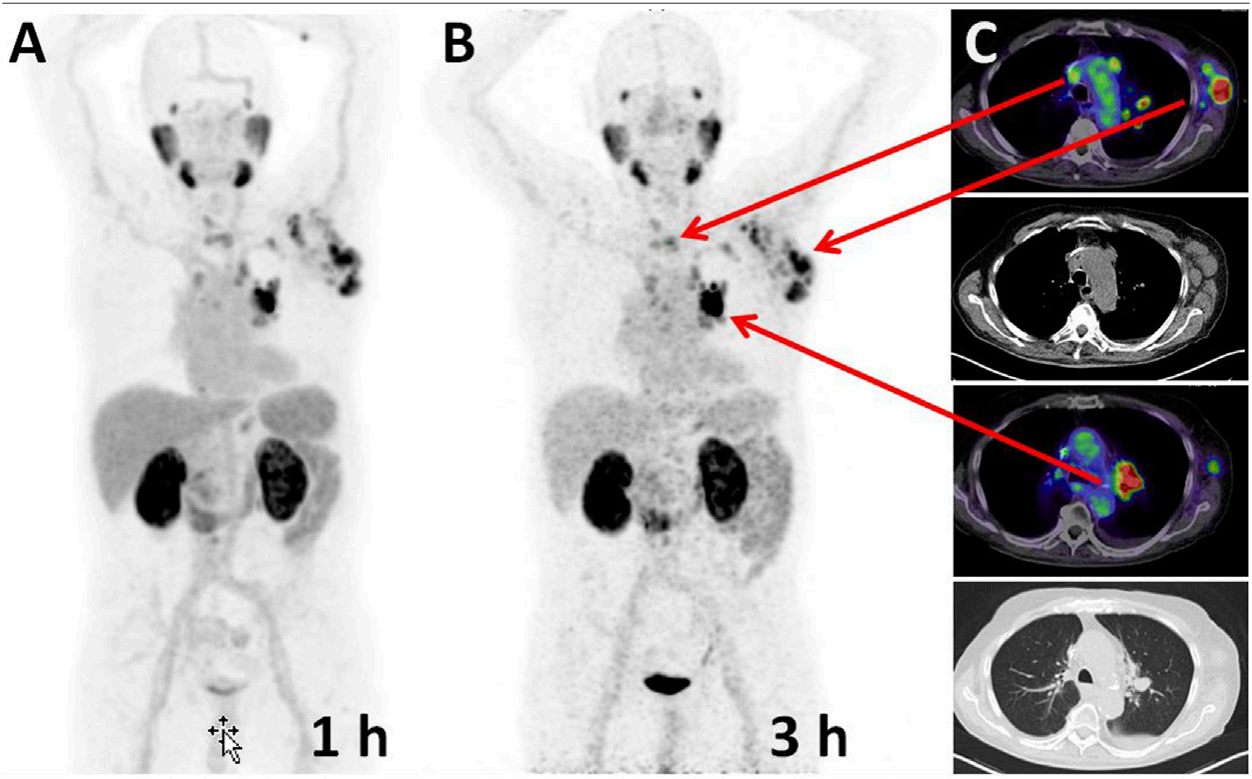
Maximum intensity projections of a PSMA-PET of a prostate cancer patient performed 1 h (A) and 3 h (B) post injection of 339 MBq/20 nmol ^68^Ga-CA028. (C) Cross-sectional slices demonstrate axillary and hilar lymph node metastases (red arrows), also delineable on the correlated CT which serves as a standard of reference.
